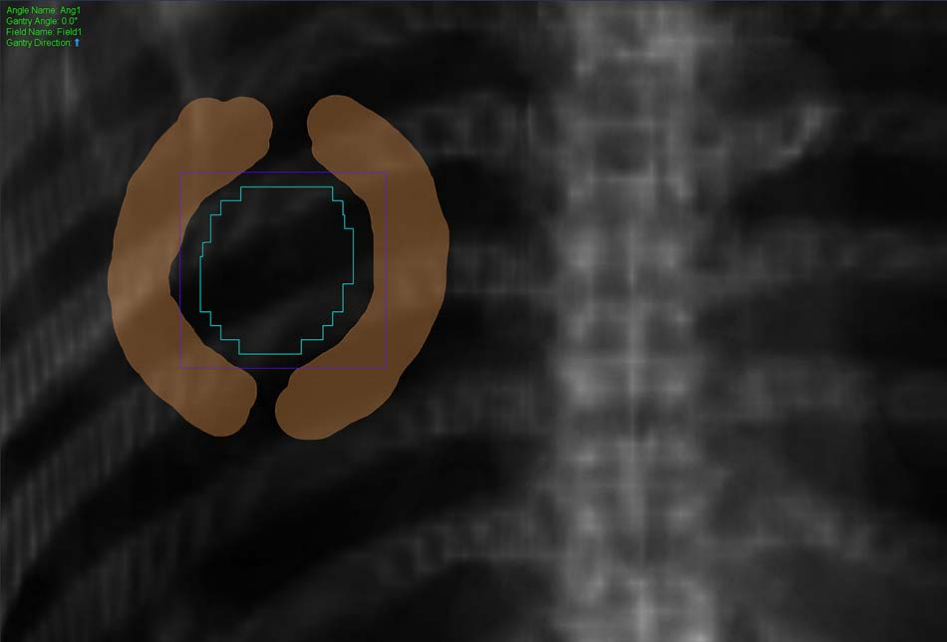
Brush tool (brown color) to modify leaf margin from each angle with digitally reconstructed radiography images in the FP mode.
